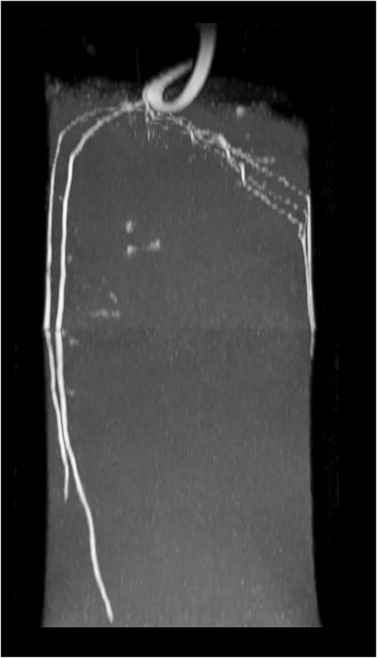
Maximum intensity projection of a MRI scan of a 14-day-old lupine root system grown in sand after dewarping and stitching (resolution 0.27 × 0.27 × 1 mm^3^).
